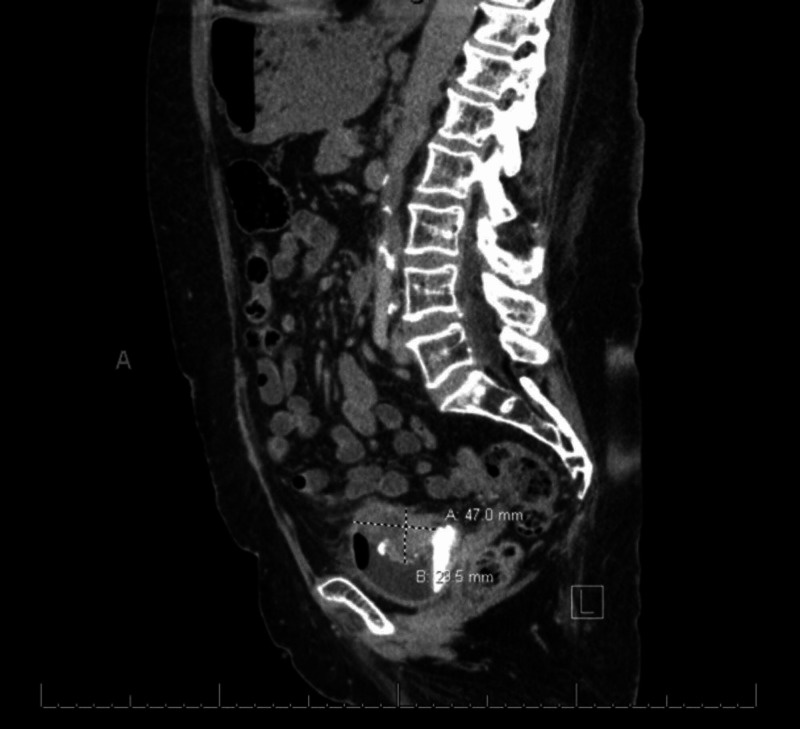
Initial CT urology during admission for gross hematuria, sagittal view demonstrating presence of calcified bladder mass. CT, computed tomography
